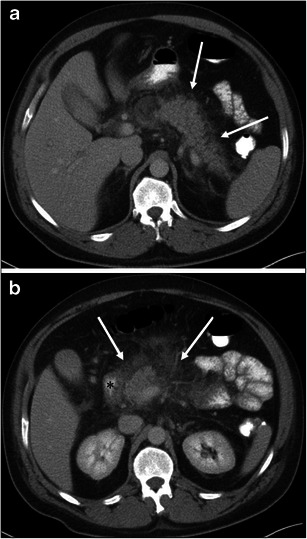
A 44-year-old male with HAE presenting with acute pancreatitis. a The axial CE-CT image shows fat stranding around the pancreatic body and tail (*arrows*). b At the level of the pancreatic head, there is more extensive peripancreatic fat stranding (*arrows*). Wall thickening of the 2nd portion of the duodenum (*asterisk*) is seen, although it could be caused by pancreatitis
